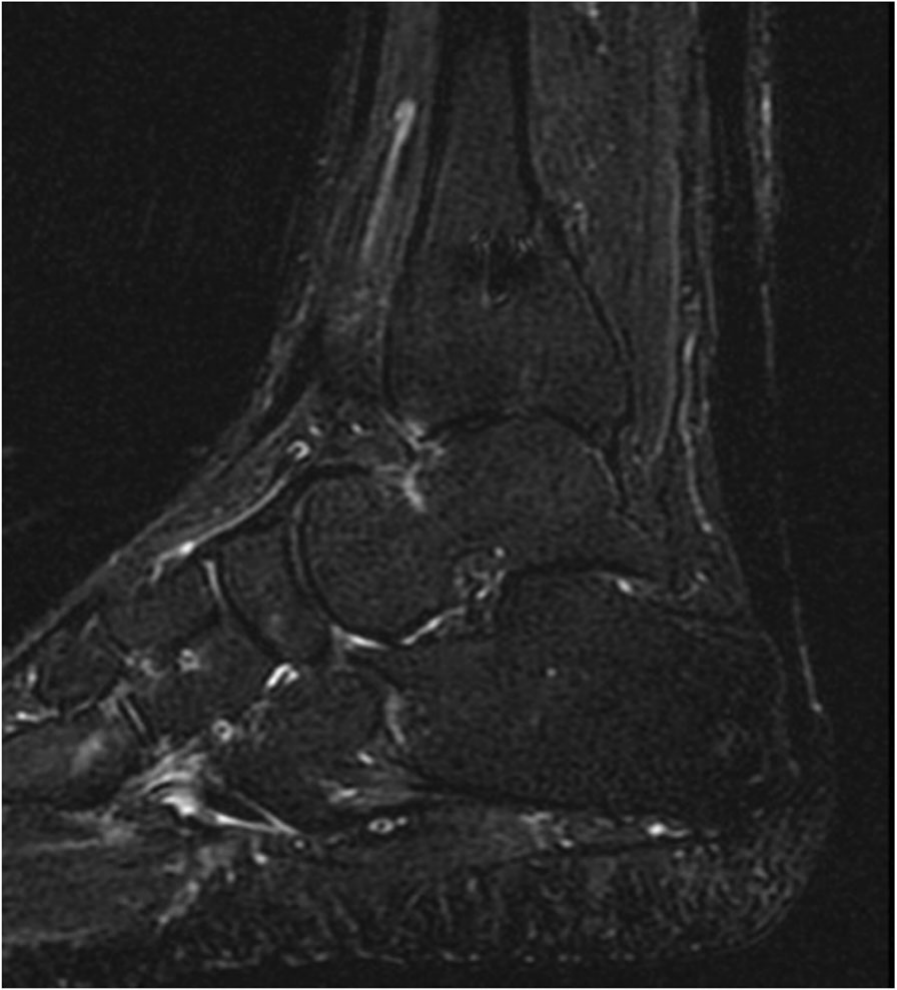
Post-operative MRI images on sagittal plane showed no recurrence and substantial continuity of the reconstruction
